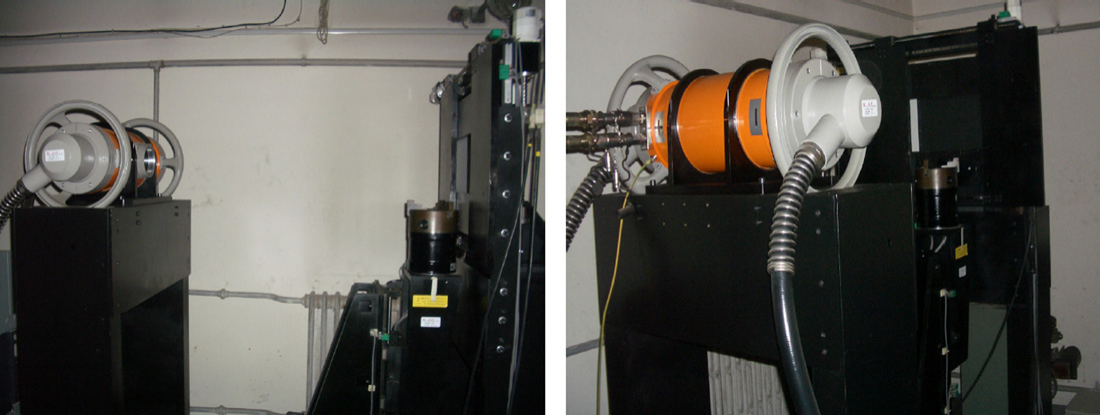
The experiment CT system. This system is composed by X-ray (GE ISOVOLT 450KV) and detector (VARIAN PaxScan2520).
